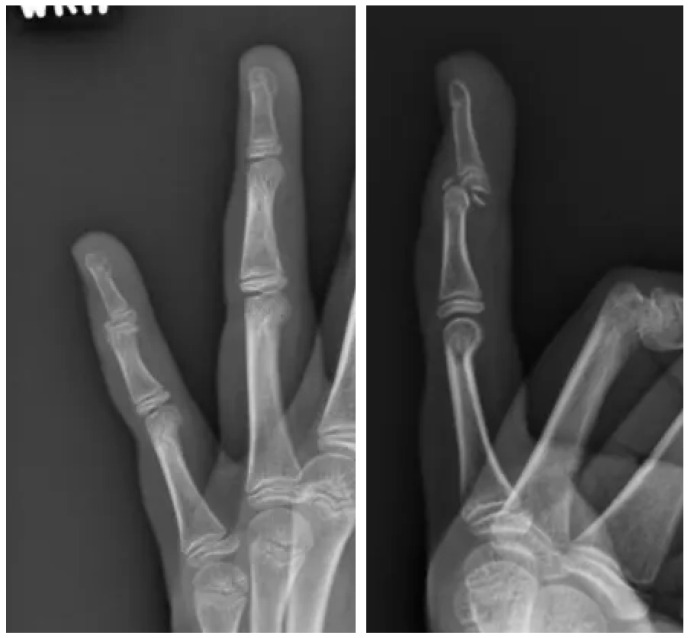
Office visit radiographs. AP and lateral views of the small finger in a skeletally immature individual demonstrating a comminuted intra-articular fracture with volar displacement of the distal phalanx.
